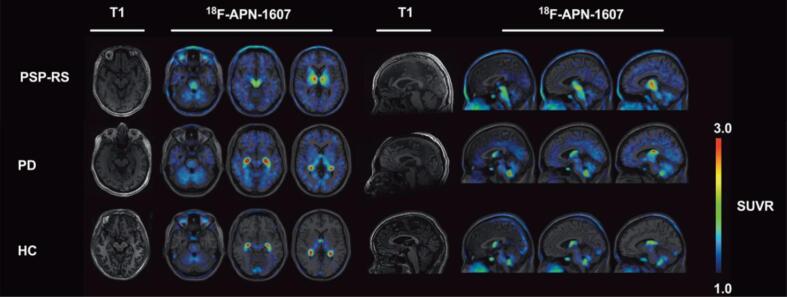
Representative [18F]-APN-1607 (or [18F]PM-PBB3) PET images superimposed on T1 MRI in PSP patients versus PD and controls. Compared to PD and HC, patients with PSP display heightened tracer binding in the midbrain, basal ganglia, subcortical nuclei, and brainstem. The colour scale represents standardized uptake value ratio (SUVR) with cerebellar cortex as the reference regions. PSP-RS = progressive supranuclear palsy-Richardson’s syndrome, PD = Parkinson’s disease, HC = healthy controls. *Figure modified from Li L, Liu FT, Li M, Lu JY, Sun YM, Liang X, Bao W, Chen QS, Li XY, Zhou XY, Guan Y, Wu JJ, Yen TC, Jang MK, Luo JF, Wang J, Zuo C; Progressive Supranuclear Palsy Neuroimage Initiative (PSPNI). Clinical Utility of 18F-APN-1607 Tau PET Imaging in Patients with Progressive Supranuclear Palsy. Mov Disord. 2021 Oct;36(10):2314*–*2323. https://doi.org/10.1002/mds.28672*. *Epub 2021 Jun 5. PMID: 34089275.*
